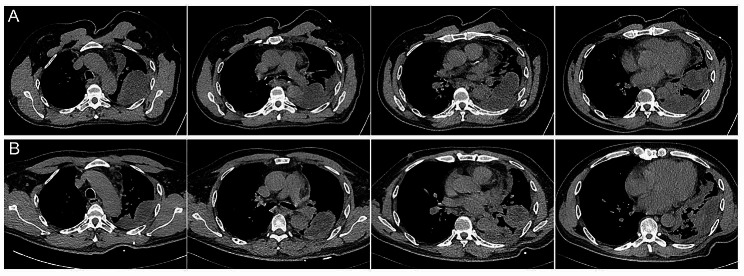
Chest CT scan showed no significant reduction of pleural effusion after chest tube placement and systemic antibiotics treatment for 8 days. (A) Chest CT findings on admission. (B) Chest CT scan 8 days after chest tube placement and systemic antibiotic therapy
